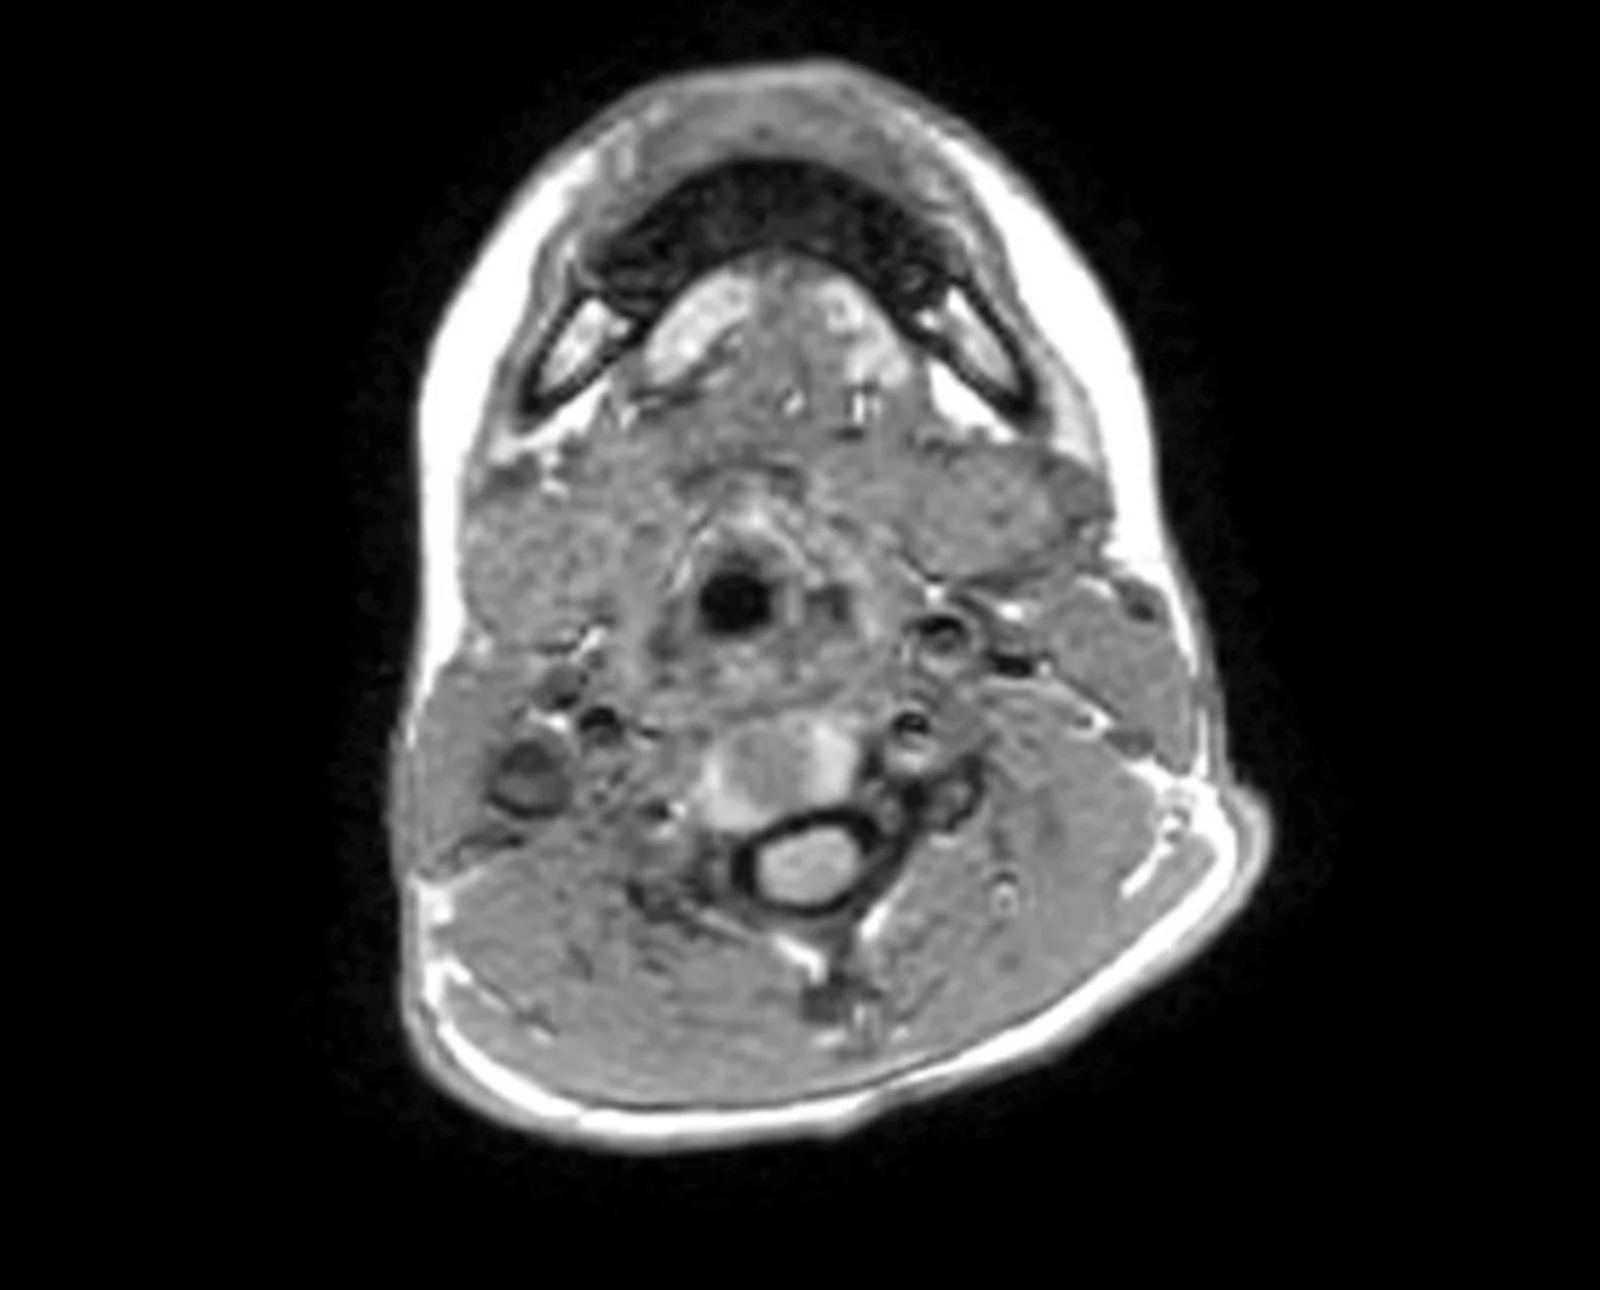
Axial T2-weighted image at the level of the upper cervical spine. Normal cervical cord signal without syrinx or cord compression. No craniocervical junction anomaly.
